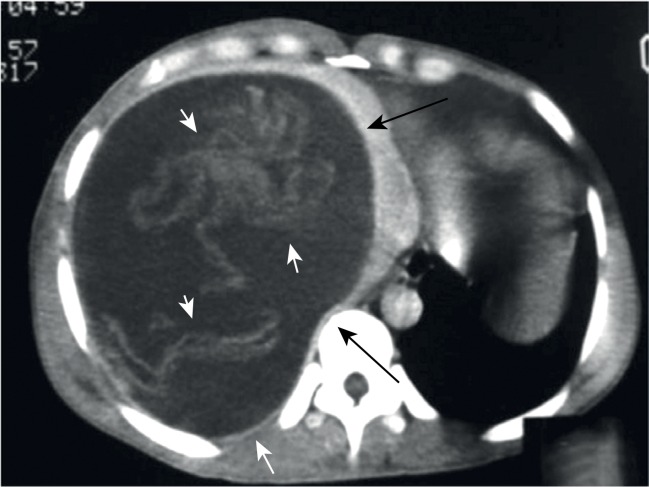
Intravenous contrast-enhanced chest CT scan with mediastinal window
